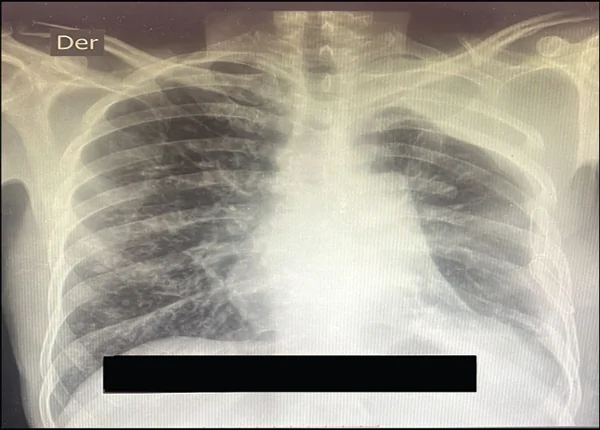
Postoperative chest radiograph demonstrating lung re‐expansion after resection of a giant pleural solitary fibrous tumor.
